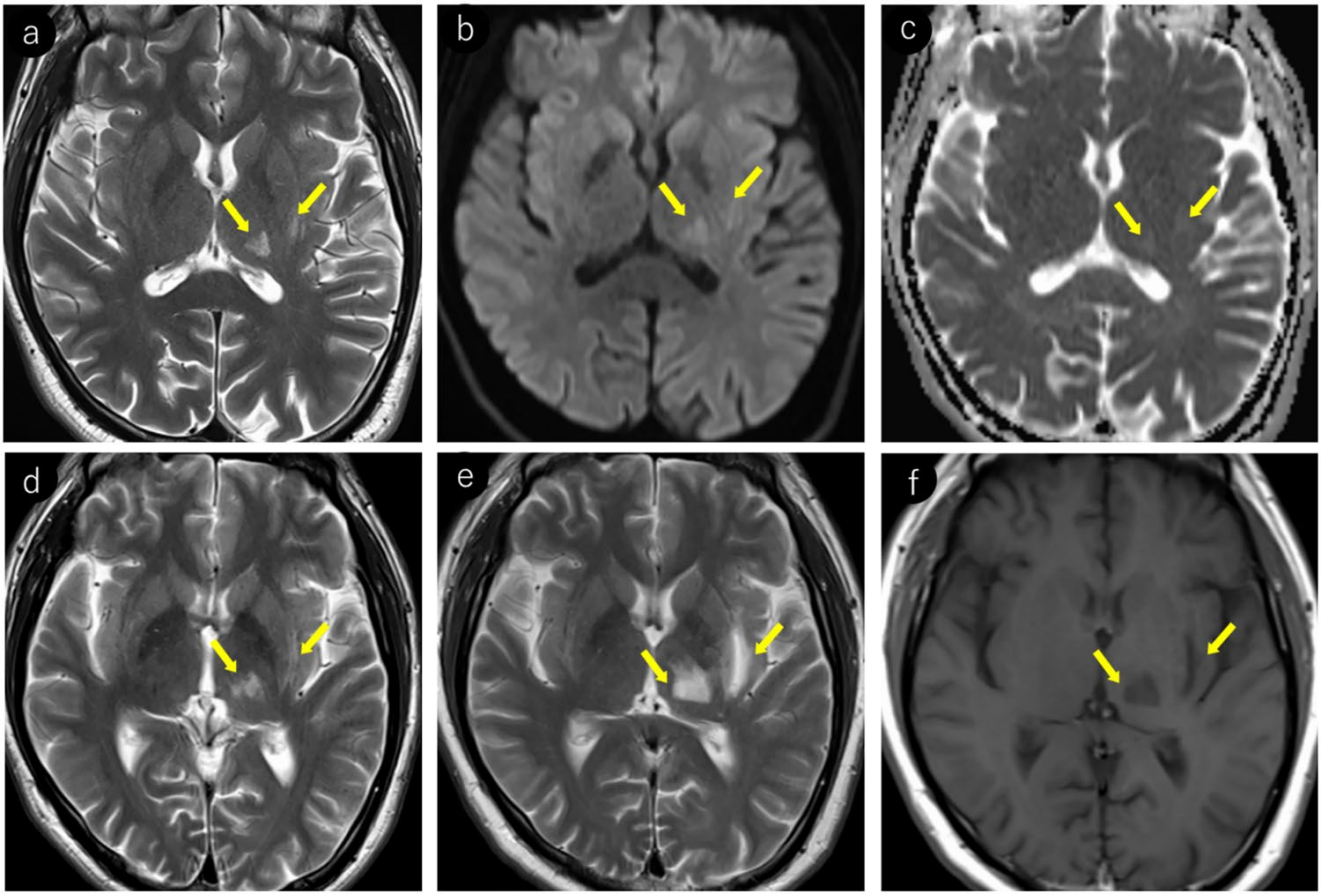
HIV-positive man in his 30 s began experiencing a sensation of heaviness in his right lower limb approximately 3 months prior, which gradually worsened. A week prior, there was a rapid loss of mobility in the right lower limb, and similar symptoms manifested in the right upper limb. Initial MRI findings (a–c): a T2-weighted image demonstrating hyperintense lesions in the left thalamus and lateral putamen (yellow arrows). b These lesions show mild hyperintensity on diffusion-weighted imaging (yellow arrows). c ADC map showing increased values without diffusion restriction (yellow arrows). Follow-up MRI findings (d–f): d 2-month follow-up imaging shows progression of the lesions with enlargement (yellow arrows). e Further progression with expansion of the lesions was observed at the 4-month follow-up (yellow arrows). f Lesions appear hypointense on T1-weighted imaging, with the lateral putaminal lesion showing a CSF-like signal intensity (yellow arrows). A cerebrospinal fluid examination confirmed the presence of the JCV, leading to a diagnosis of PML
